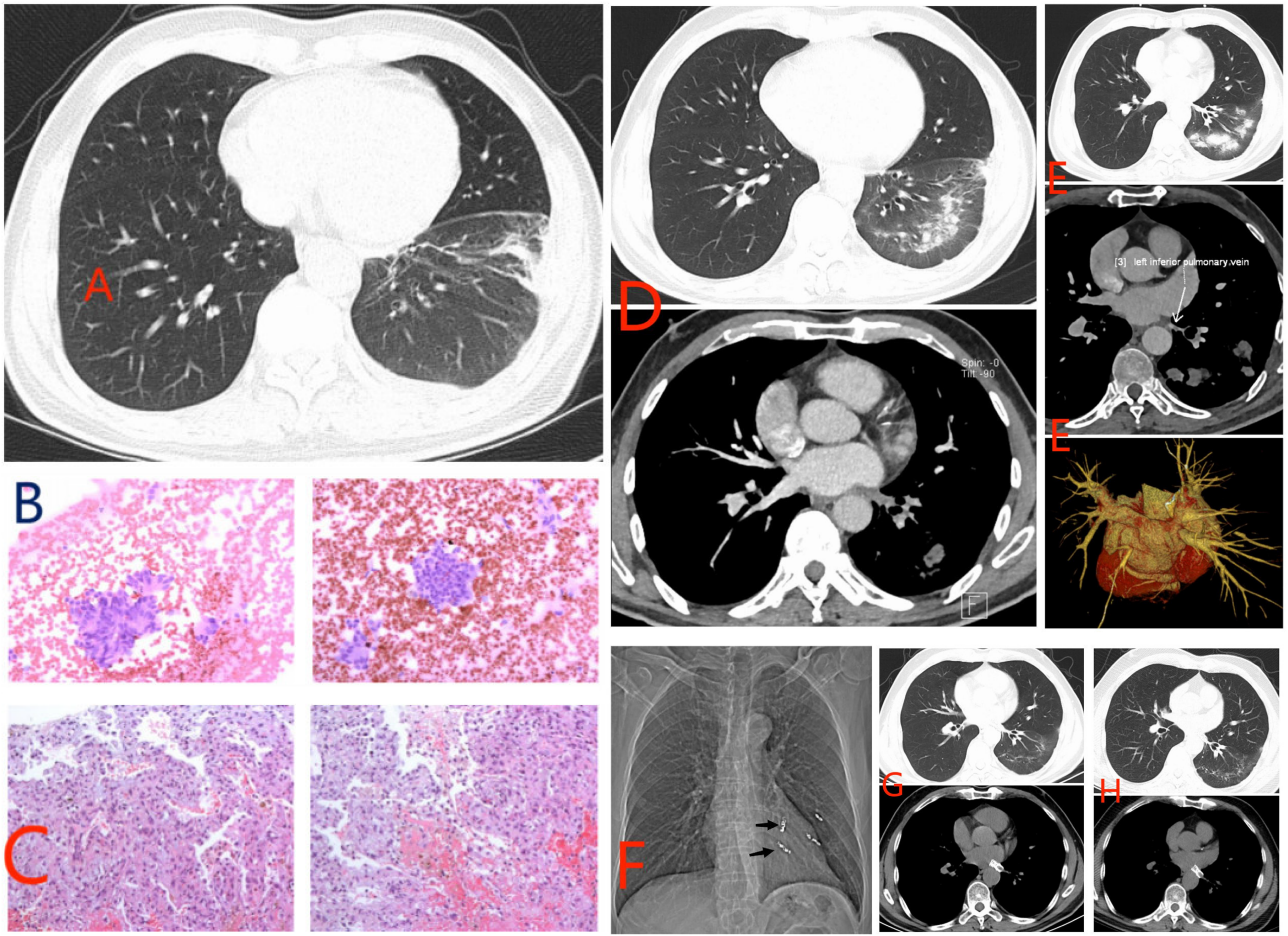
Information on ancillary tests for patients in clinical care. (A) Inflammation of the lower lobe of the left lung and pleural effusion. (B) Cytological examination. (C) Percutaneous lung biopsy. (D) Pulmonary artery CT scan. (E) Pulmonary vein contrast-enhanced CT scan shows left lower pulmonary vein occlusion. (F) Bleeding artery embolization. (G) Lung CT shows improvement in condition. (H) Review lung CT on March 1, 2024.
